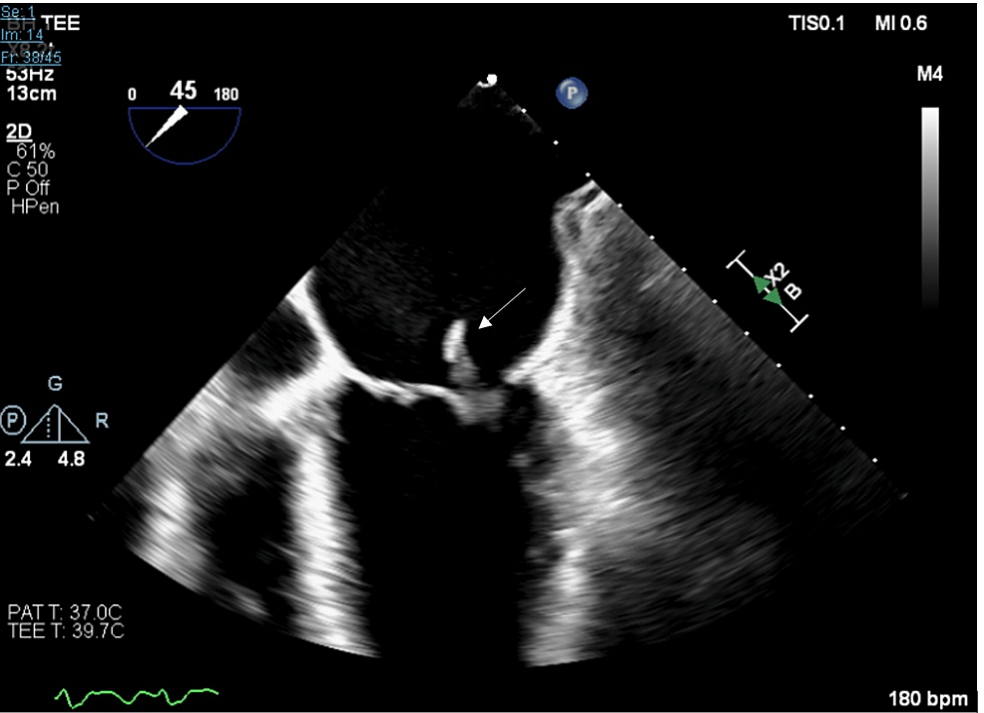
Transthoracic echocardiogram showing large mobile vegetation on the left atrial side of the posterior mitral valve leaflet.
